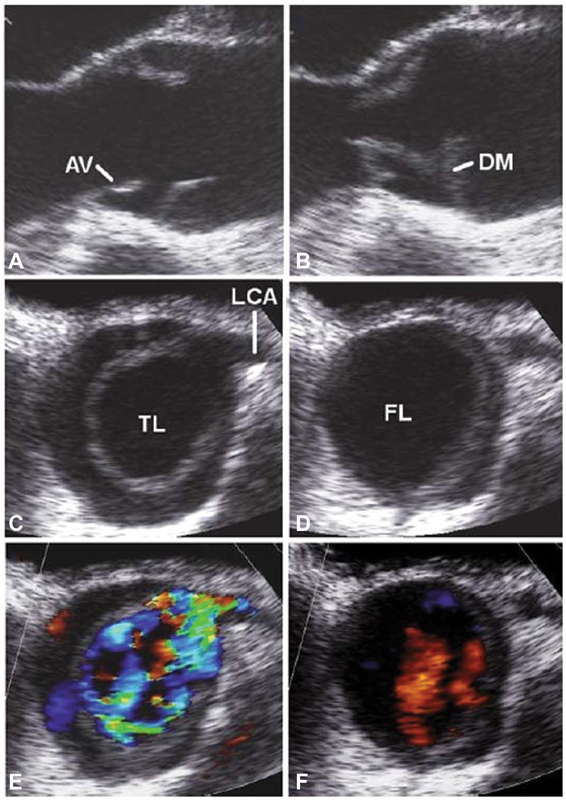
Intraoperative transesophageal echocardiography. Systolic (
